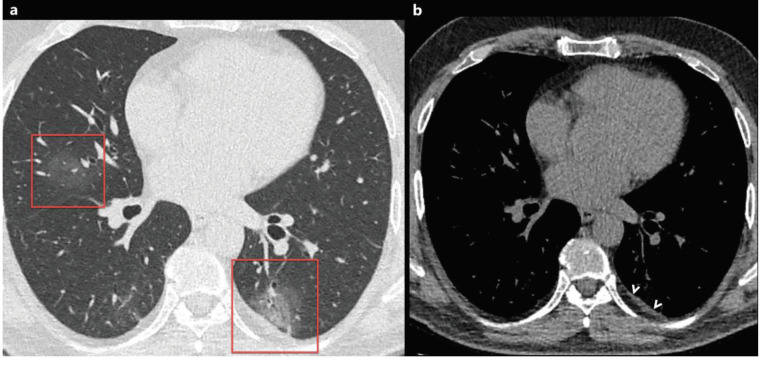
A 56-year-old man with COVID-19. Axial chest CT image with lung window settings shows bilateral focal ground-glass opacities in both lungs (a, red frame). Chest CT image with mediastinum window settings shows focal pleural thickening in the left lower lobe, near the focal ground-glass opacity (b, arrowheads).
